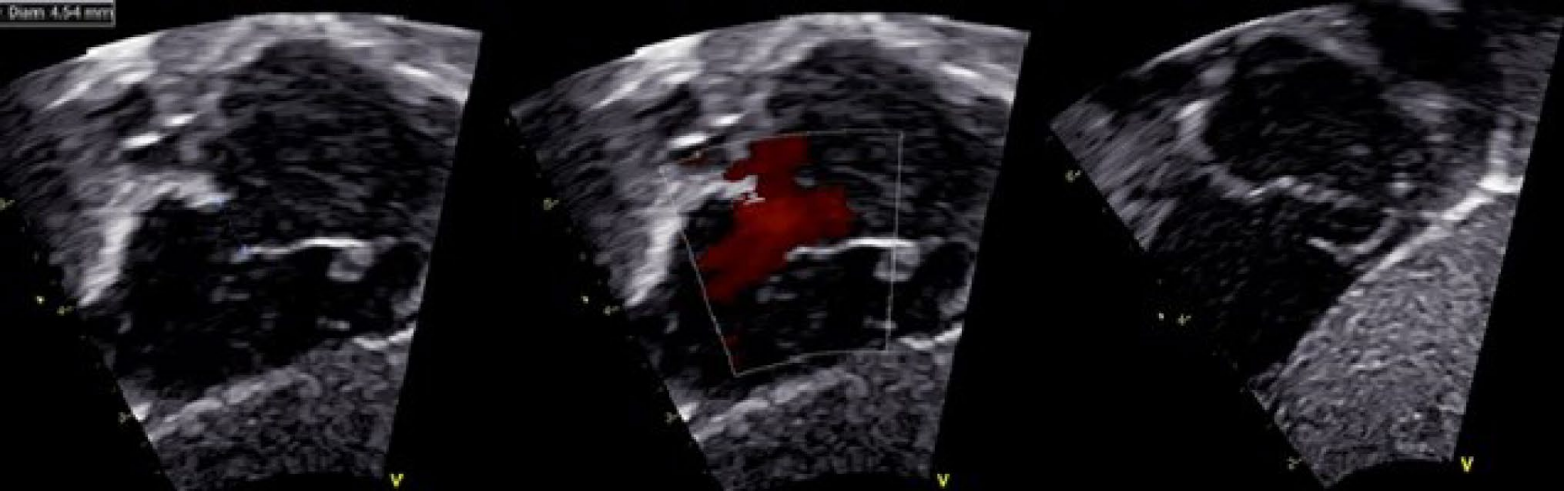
Three transthoracic echocardiographic images of the atrial septum in two subxiphoid views where the atrial septum is best visualized using cross-sectional images and color Doppler. A sweep through the atrial septum was performed to investigate the septum thoroughly and to capture a possible IAC
